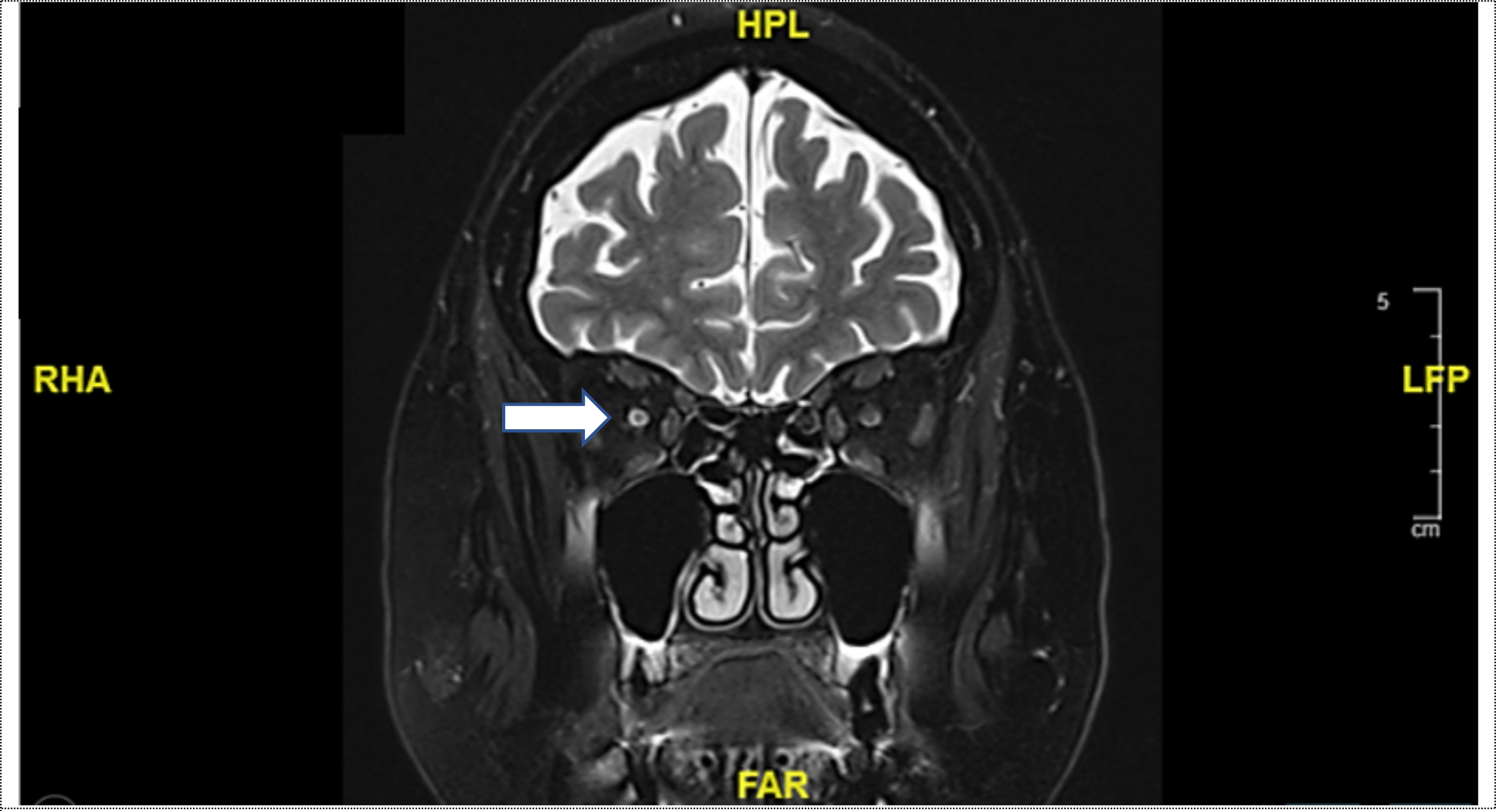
Coronal T2-weighted magnetic resonance imaging (MRI): Increasing cerebrospinal fluid (CSF) signal along the left optic nerve with continued minimal contrast uptake (white arrow).
